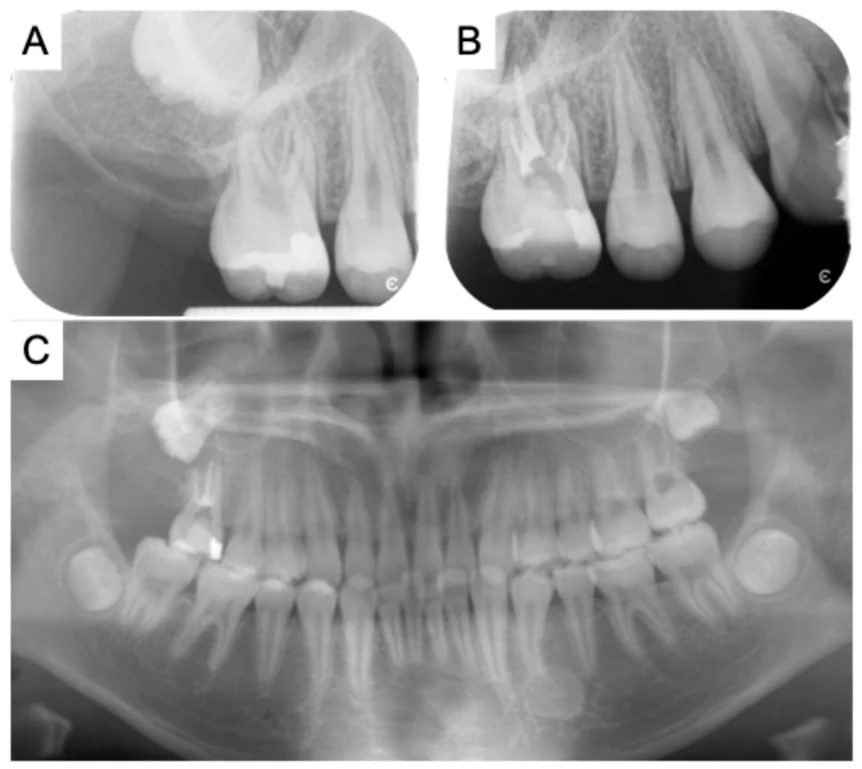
Radiographic examination before and after root canal treatment of the first molar. Based on the clinical diagnosis of a maxillary cyst, provisional pulpectomy of the first molar was initially performed in cooperation with the Department of Periodontology (A,B). By this time, the patient’s cooperation had improved, and physical restraint was no longer required. In addition, panoramic examination at 13 years and 2 months of age showed no significant changes in the cyst (C). On the following day, cystectomy and removal of the impacted second and third molars were performed under general anesthesia in the Department of Oral Surgery. An incision was made in the buccal gingiva in the region of the second premolar and first molar, and the mucoperiosteal flap was elevated. A bone defect was observed; the surrounding bone was removed, and the lesion was extracted en bloc with the second molar. The adjacent third molar was subsequently extracted, and the site was curetted and sutured.
